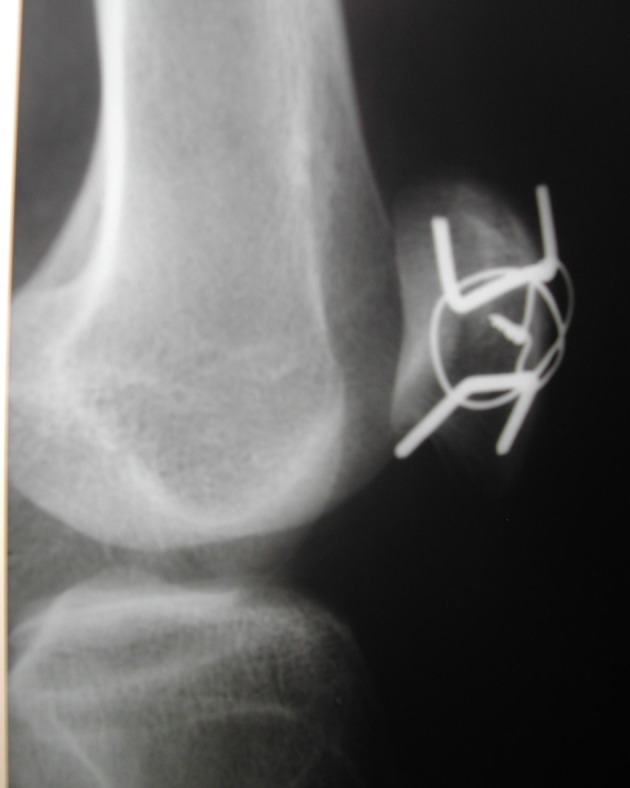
Seven days postoperatively, oblique radiograph demonstrated better articular surface without joint gaps.
